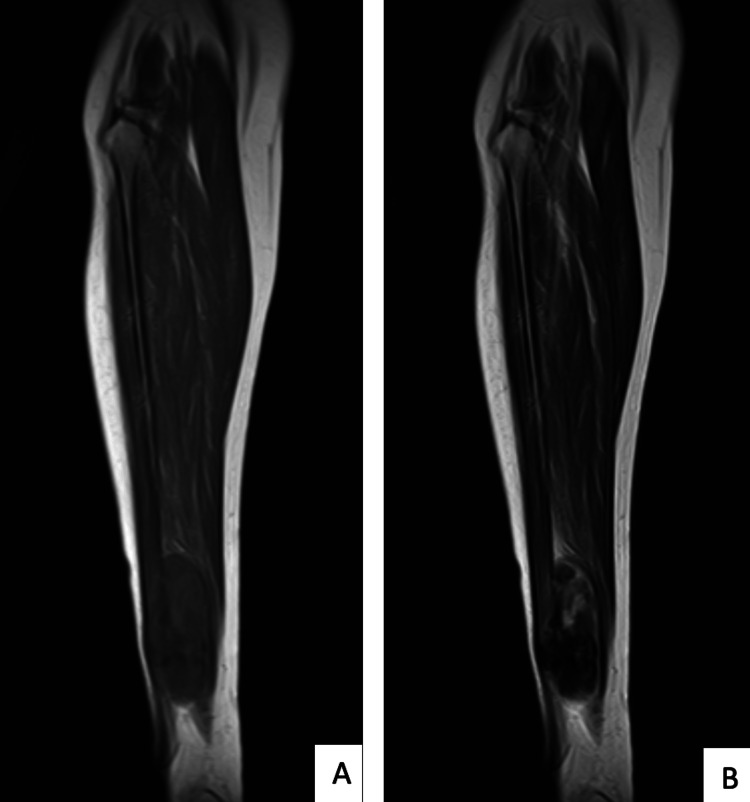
Pre-operative MRI image in coronal cut showing homogeneous isointense in a T1-weighted signal (A) and a heterogeneous hyperintense, well-defined oval-shaped mass on a T2-weighted signal with avid post-contrast enhancement (B) MRI, magnetic resonance imaging
